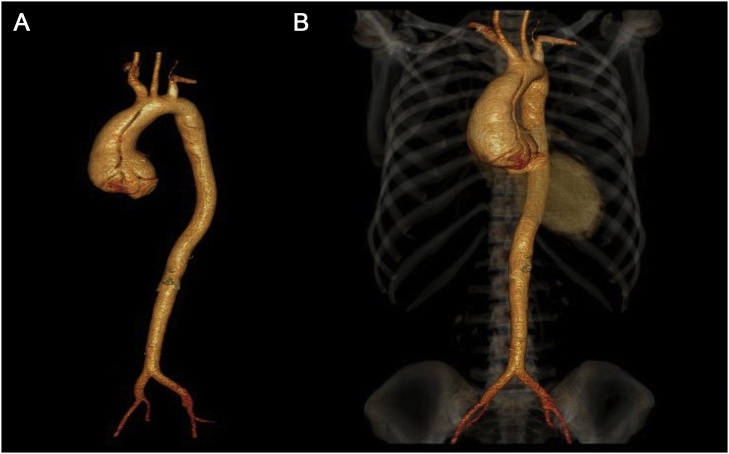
A and B: CT Aortogram shows dilated aortic root and ascending aorta which was 45 mm and 58 mm respectively and a dissection flap from just above the Left Main Coronary Artery (LMCA) ostium and involving the proximal segment of Right coronary artery (RCA) extending to the proximal aortic arch.
